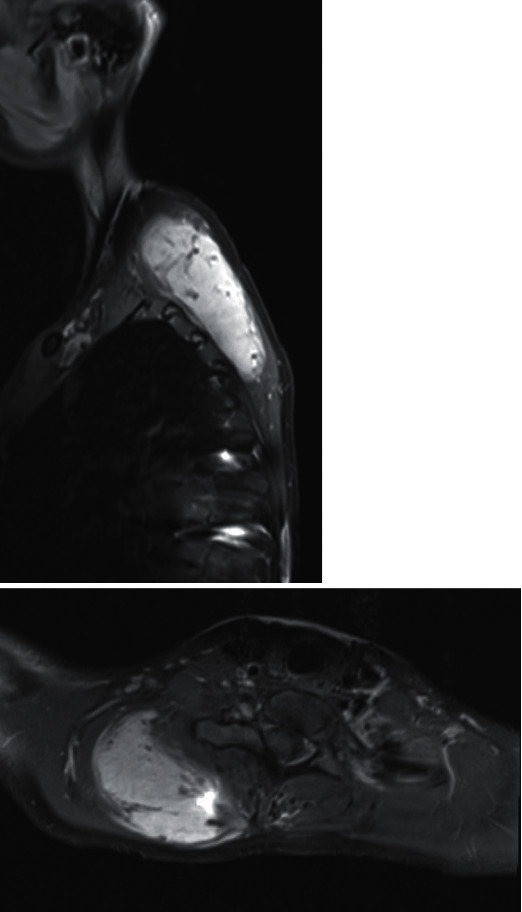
Right parasagittal and axial sections of T1-weighted MRI demonstrating increased size of contrast-enhancing paraspinal mass.
